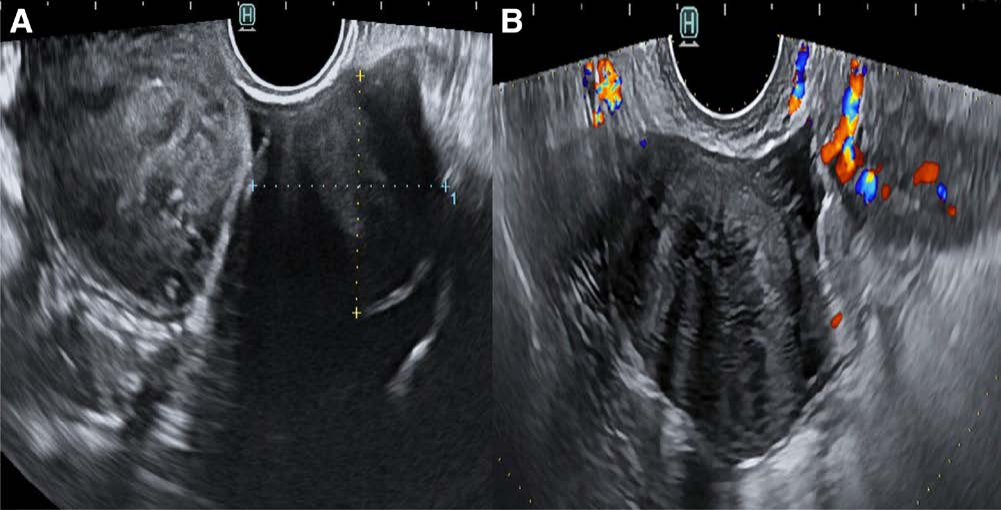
Transvaginal ultrasound findings. (A) A well-defined, solid, oval, and hypoechogenic mass with stripy shadows measuring 41 × 40 mm was seen in the left adnexa. (B) There was no vascularity in the mass on color Doppler.
